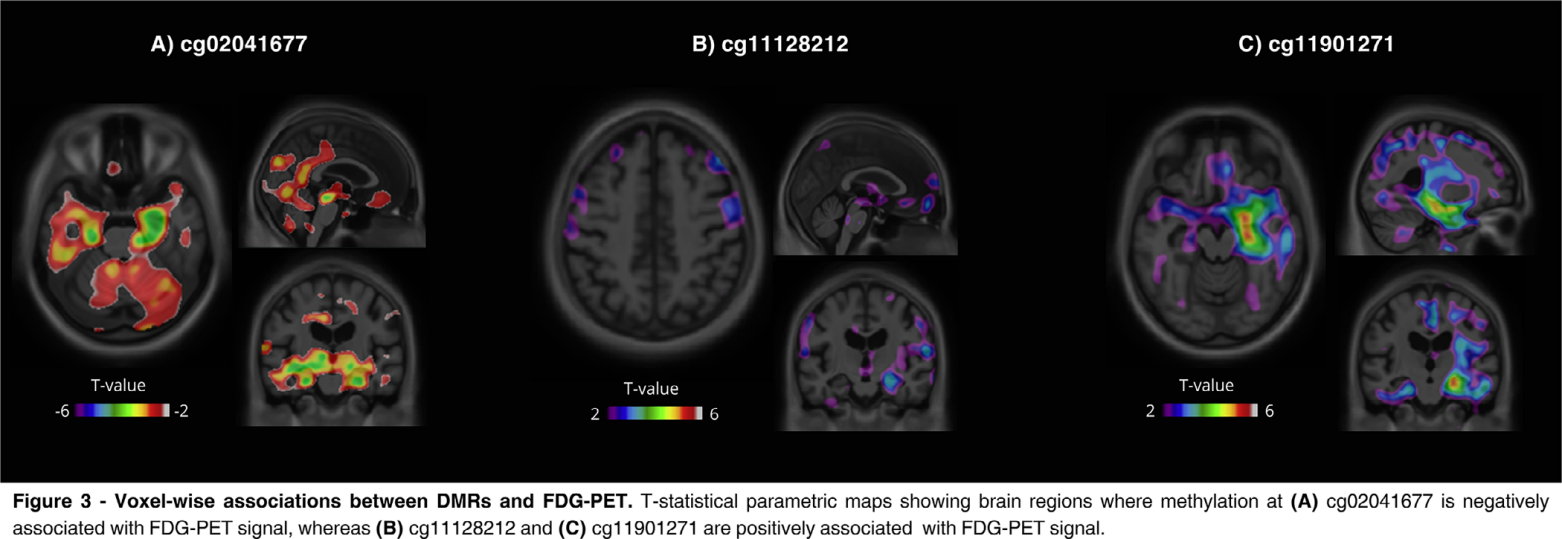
# Associations between peripheral blood DNA methylation and FDG‐PET signal in AD individuals
